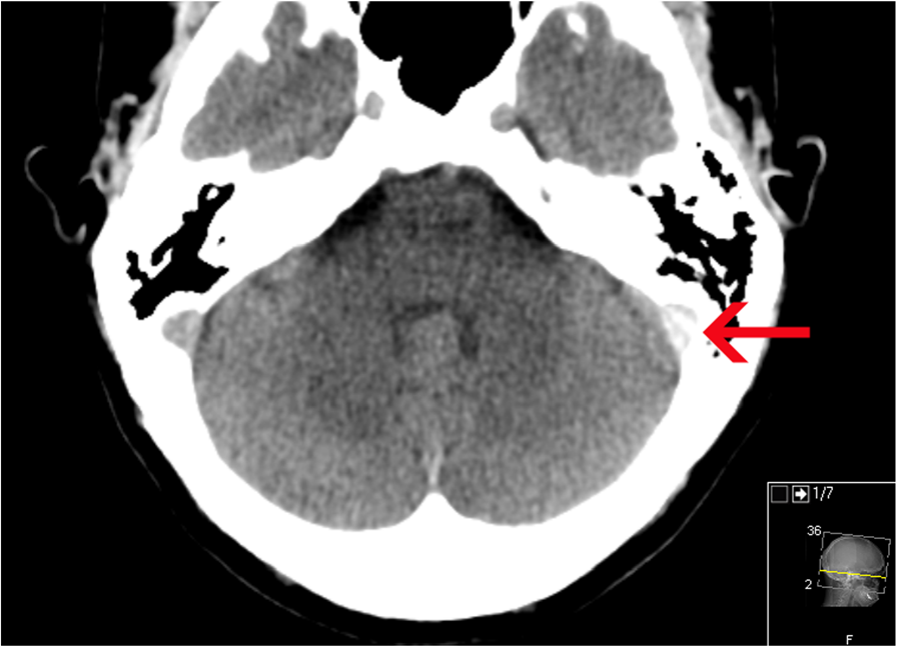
Axial reformatted 5 mm nonenhanced CT of suspected thrombus in the left sigmoid sinus marked by a red arrow (window width 90 window level 40). Note the normal HU value in the corresponding right sigmoid sinus
